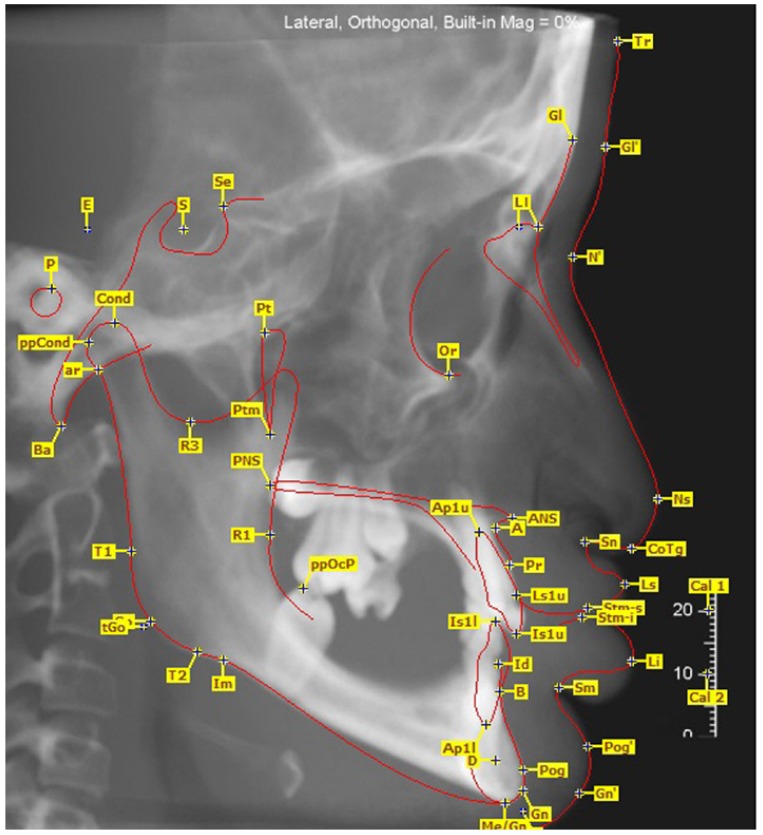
Lateral cephalometric image obtained with CBCT and the reference points.
